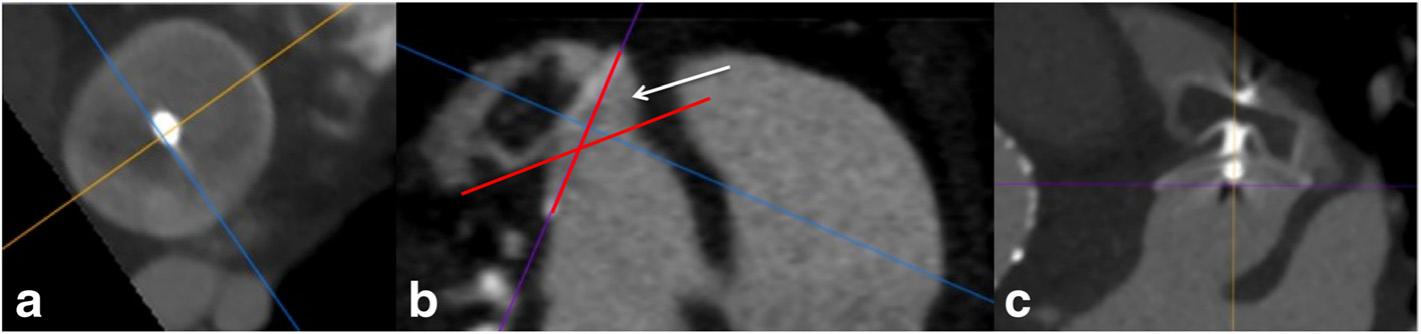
LOVE - axial (panel a), − sagittal (panel b), and - coronal (panel c) reformations of a 83-year-old female patient who underwent cardiac CTA 9 months after implantation of the ACP® LAA occlusion device. Panels a, b and, c demonstrate no peri-device leak. Panel b shows incomplete lobe coverage with a small residual LAA lobe. The uncovered LAA lobe is seen between the angle of the sealing part of the ACP device (proximal disk) and the plane of the LAA ostium (angle in between *red lines*, marked by *white arrow*). The contrast filling of the LAA in combination with the clear absence of a peri-device leak reflects indirectly in-complete endothelialization of the ACP® LAA occlusion device
